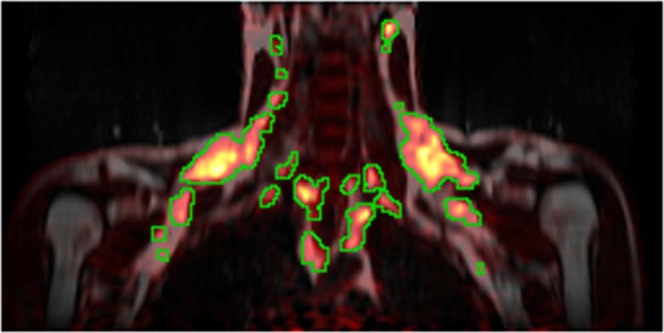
Coronal slice showing multiple BAT depots. MRI-derived fat signal image from the dual-echo scan in grayscale with the PET-derived GUR superimposed using the heat colourmap. The green lines delineate the areas over which the total BAT GUR was summed.
